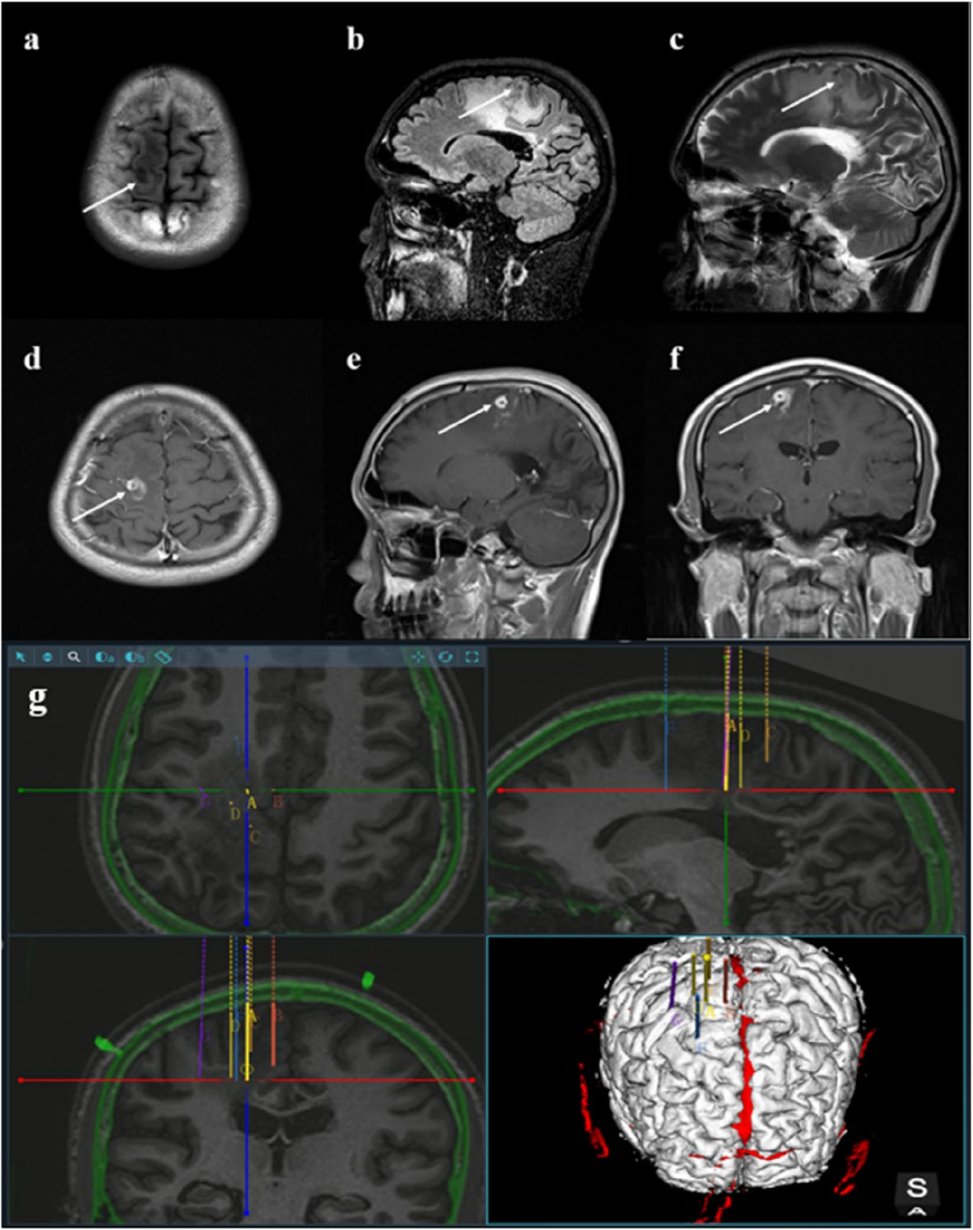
Preoperative MRI images in axial (a) T1-weighted, sagittal (b) T2/FLAIR-weighted, and sagittal (c) T2-weighted scans. Preoperative contrast-enhanced T1-weighted MRI images in the axial (d), sagittal (e), and coronal (f) planes are presented. Neurosurgery robot-assisted frameless stereotactic technology was applied to implement the surgical strategy for lesion biopsy and resection in functional areas (g)
